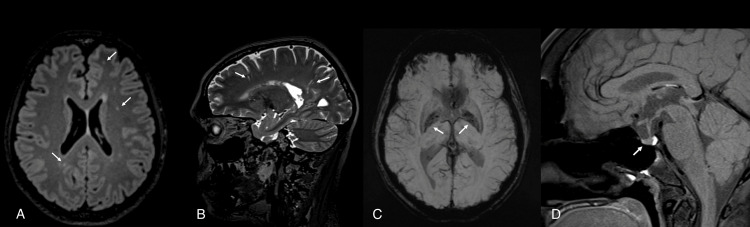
Brain MRI of Patient 1 (A) Multiple and bilateral faint FLAIR/T2 hyperintensities predominantly involving the frontal and parietal lobes; (B) sagittal T2 image showing multiple prominent perivascular spaces; (C) susceptibility-weighted imaging sequences showing mild to moderately increased mineralization/iron deposition in the globus pallidus and putamen for the patient's age; (D) the anterior pituitary measures 3.3 mm in height and is small for the patient's age.
